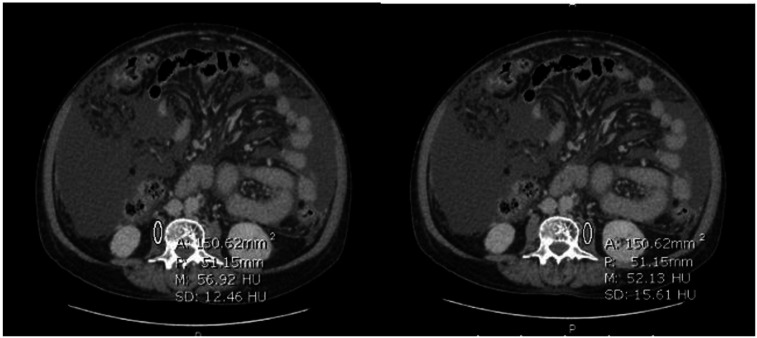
Computed tomography image for assessment of muscle density using region of interest.
